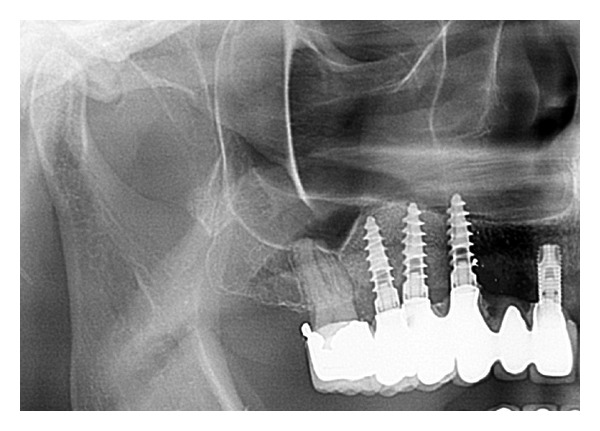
Case 3: panoramic X-ray after final prosthetic treatment after 11 months.
